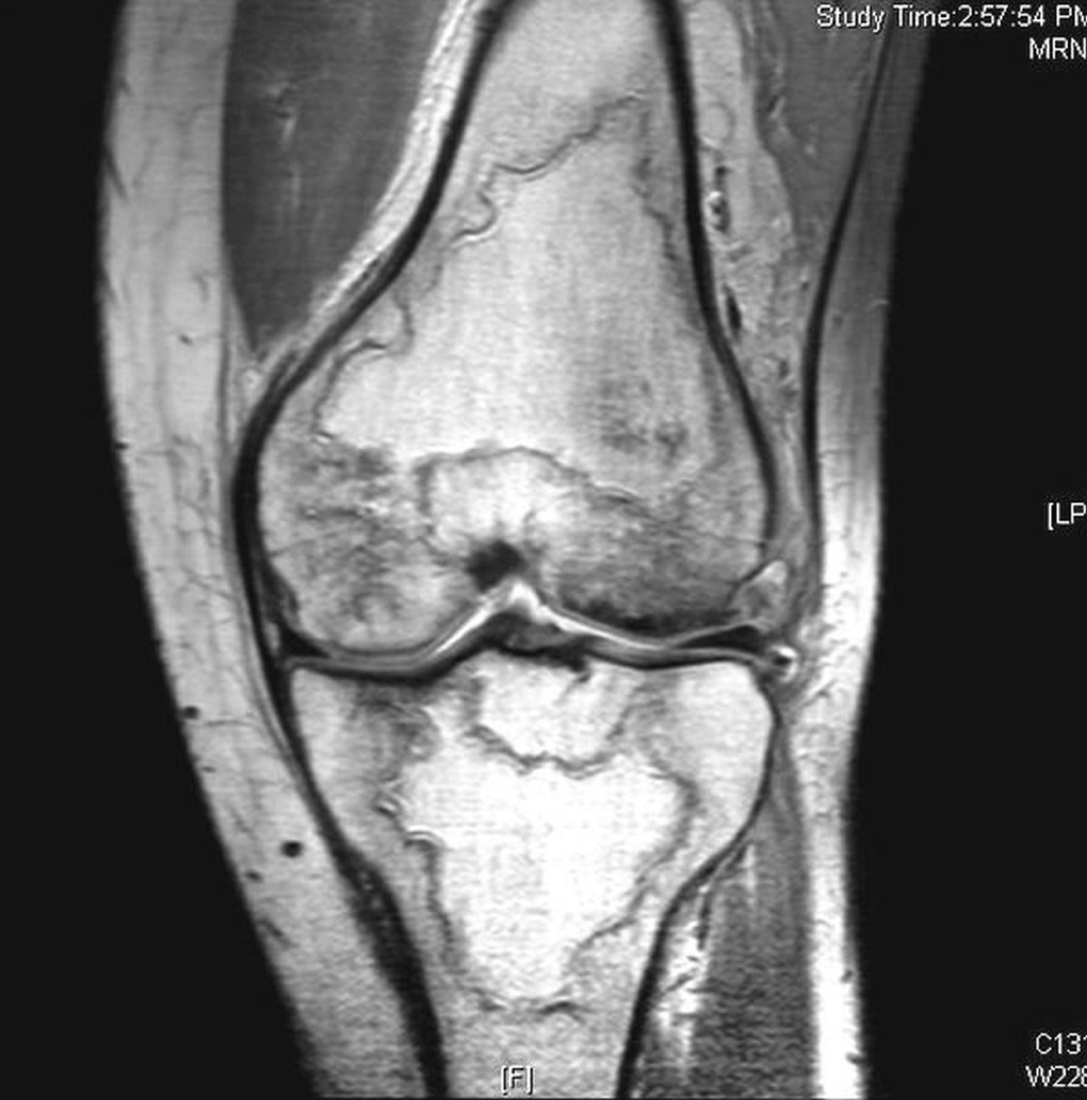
MRI of the knee showing multiple infarcts
